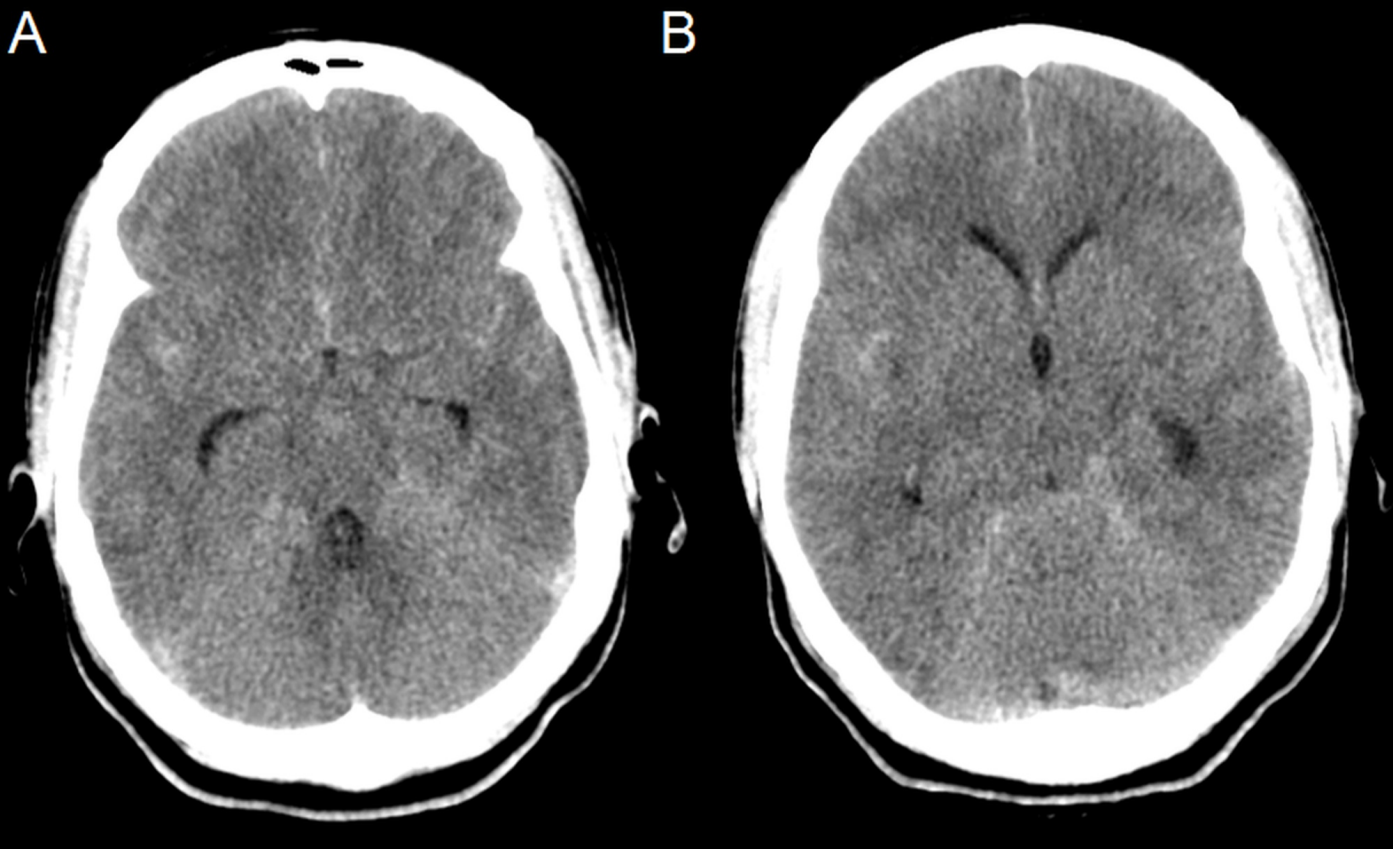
Axial pre-operative CT head scans (A and B) from outside institution showing subarachnoid hemorrhage and hydrocephalus
